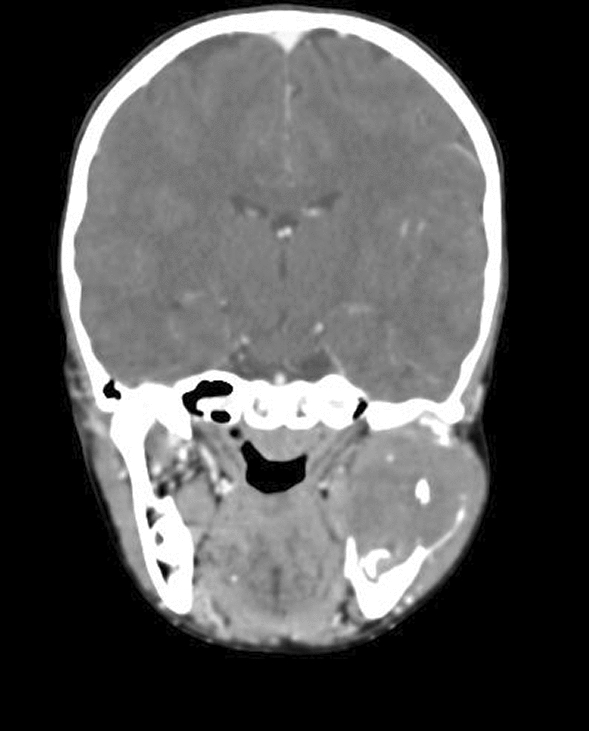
Coronal section of the contrast enhanced computed tomography scan showing gross destruction of the mandible
